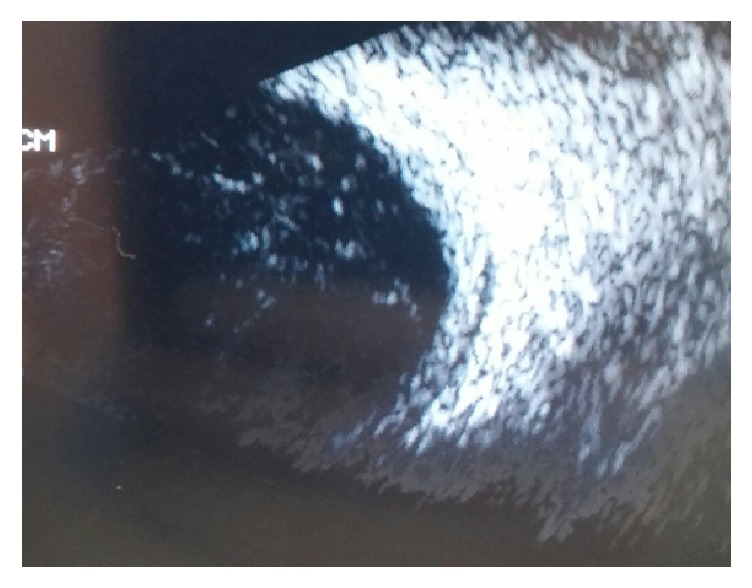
Ultrasound of the right eye. Ultrasound of the right eye shows a thickened retina and debris within the vitreous humor. These findings are consistent with inflammation and purulent material within the eye.
